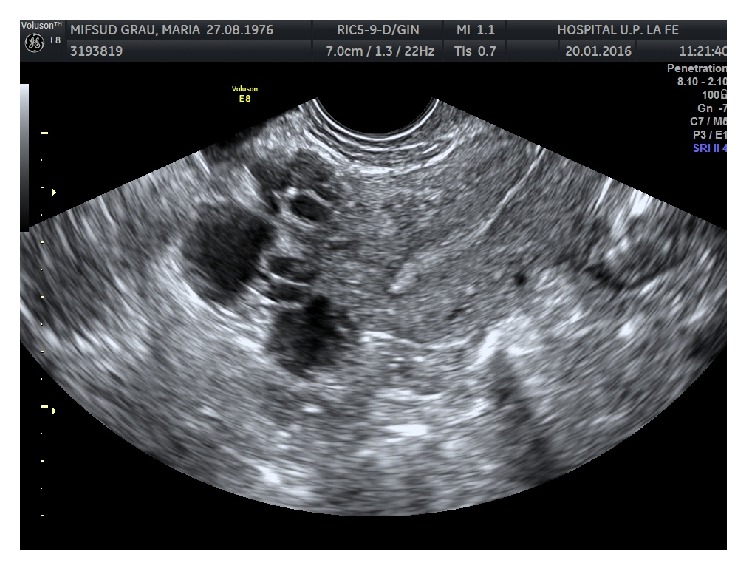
Transvaginal ultrasound image of the cystic endosalpingiosis lesions 3 months after the cesarean section. The fundus and part of the uterine body were still covered with multitude of cyst-like structures resembling hydatids of Morgagni. The endosalpingiosis lesions did not disappear after pregnancy.
